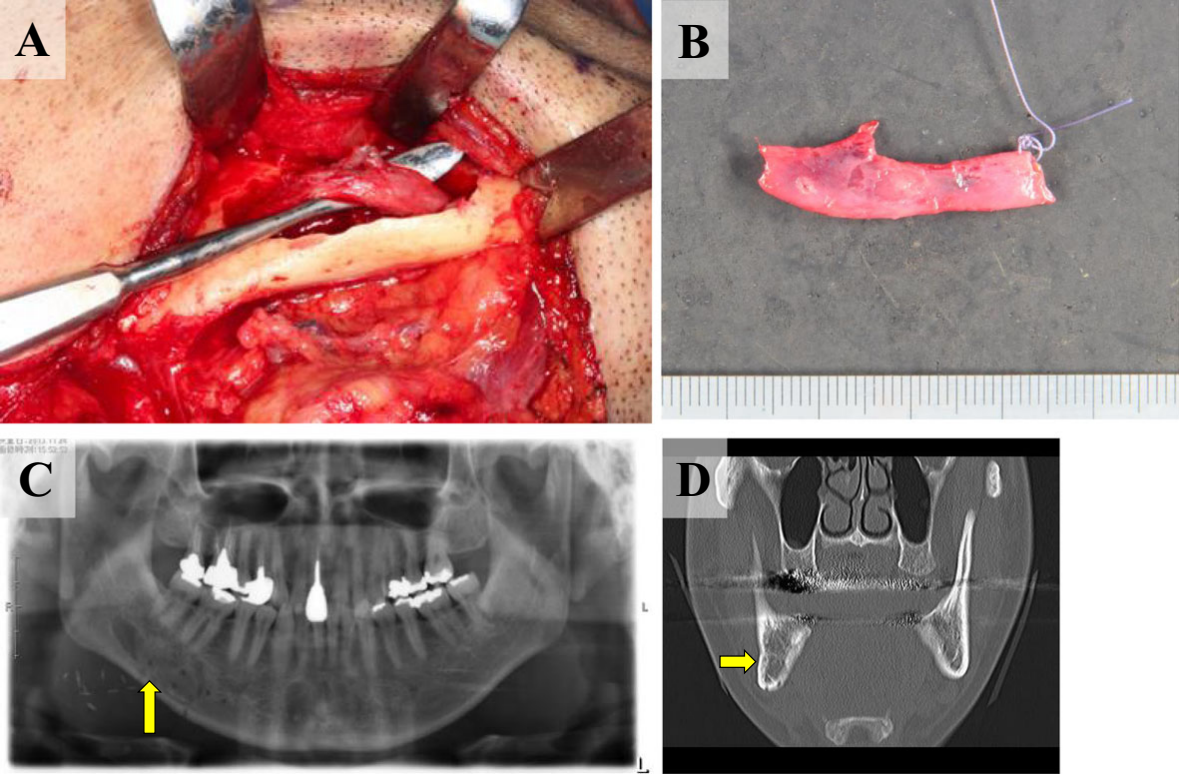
a Intraoperative findings. A cystic tumor of 3.2 × 1.0 cm was observed macroscopically following the inferior alveolar nerve. b Extirpation of tumor findings. The surrounding inferior alveolar neurovascular bundle was ligated and cut and removed as a lump. c, d Postoperative panoramic X-ray and X-ray CT findings. The radiopacity of the excised cavity was enhanced, and hyperostosis was confirmed
